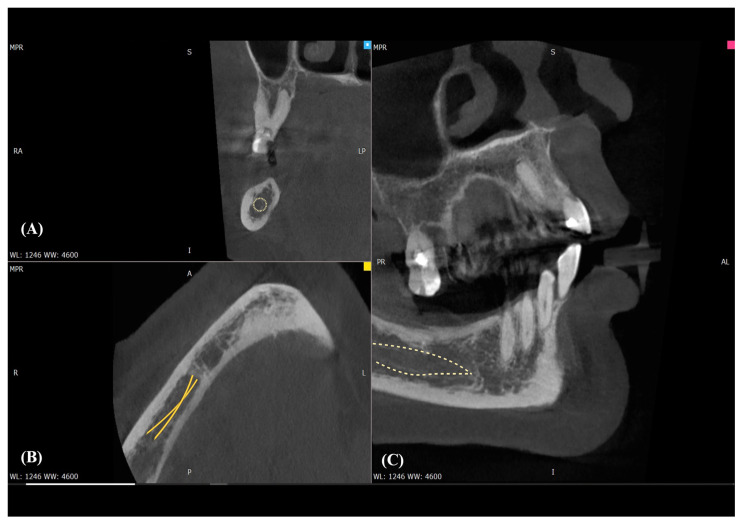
CBCT multiplanar visualization of the mandibular canal (MC). (A) Axial view showing the corticated cross-section of the mandibular canal (yellow dotted circle). (B) Sagittal reconstruction demonstrating two parallel corticated tracts suggestive of a bifid configuration of the mandibular canal (yellow lines). (C) Coronal reconstruction outlining the course of the main mandibular canal within the mandibular body (yellow dotted outline).
